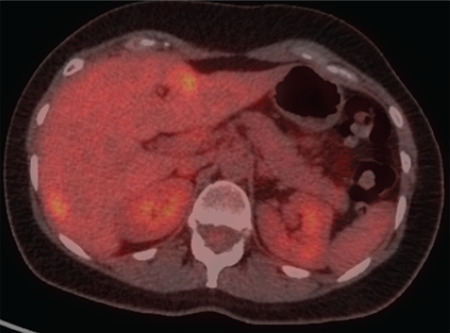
A baseline PET/CT of a patient with 2 liver metastases from breast cancer, who was treated with RFA. Pre-treatment axial fused slice on the level of liver segments 2 and 7, showing metabolically active liver lesions respectively with SUV_max_ 5.2 and SUV_max_ 4.3 PET/CT: Positron emission tomography/computed tomography, RFA: Radiofrequency ablation, SUV_max_: Maximum standard uptake value
